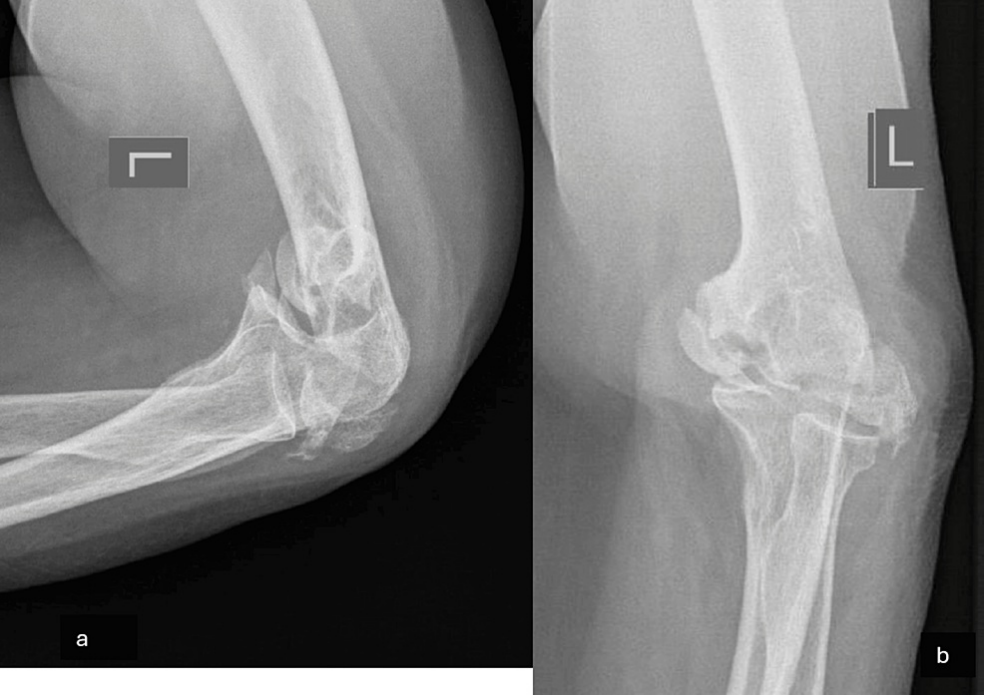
(a) Lateral elbow X-ray demonstrating the condyles. (b) Anteroposterior distal humerus X-ray demonstrating the non-union of the condyles.
